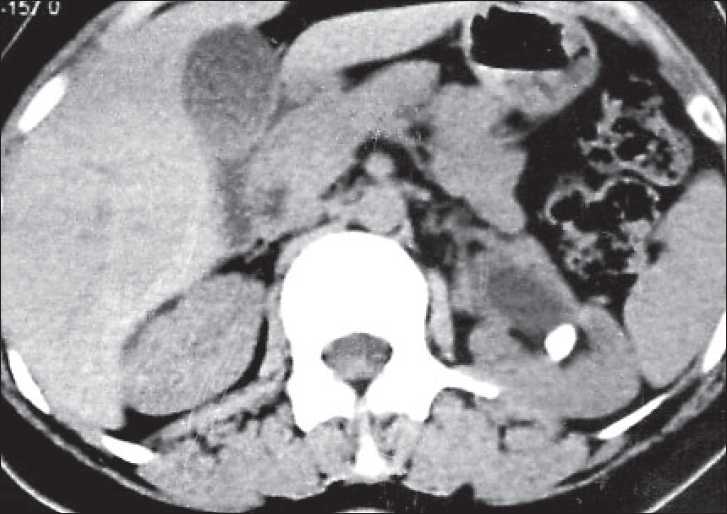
Non-contrast computed tomography showing left renal pelvic calculus
